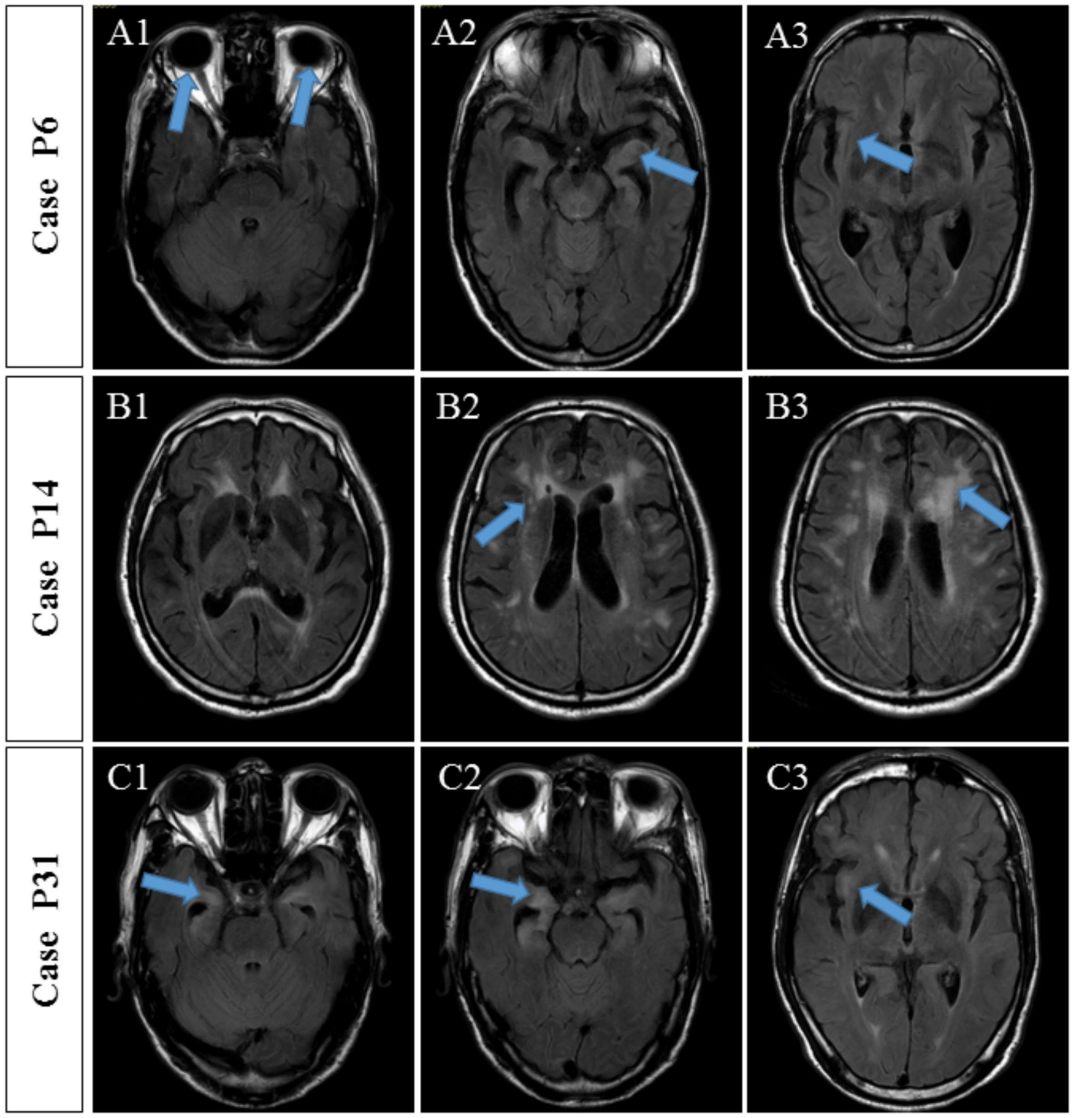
MRI findings of three patients with autoantibody-positive neurosyphilis. (A) Case P6, MRI showed multiple abnormal signal shadows in the bilateral temporal cortex and subcortex. (B) Case P14, MRI showed multiple ischemic lesions in the frontotemporal parietal lobe and the lateral ventricle and brain atrophy. (C) Case P31, MRI showed multiple abnormal signals in the bilateral frontal, temporal, and insular lobes, indicating inflammatory lesions and brain atrophy.
